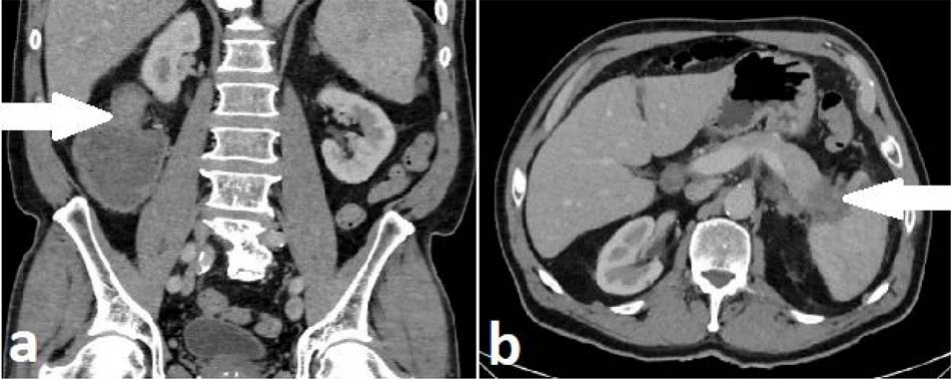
Case B. Portal venous phase CT scan of the abdomen. Coronal view of short segment hepatic flexure thickening (arrow) with proximal large bowel obstruction (a) and axial view of soft tissue mass (arrow) at tail of pancreas (b).
